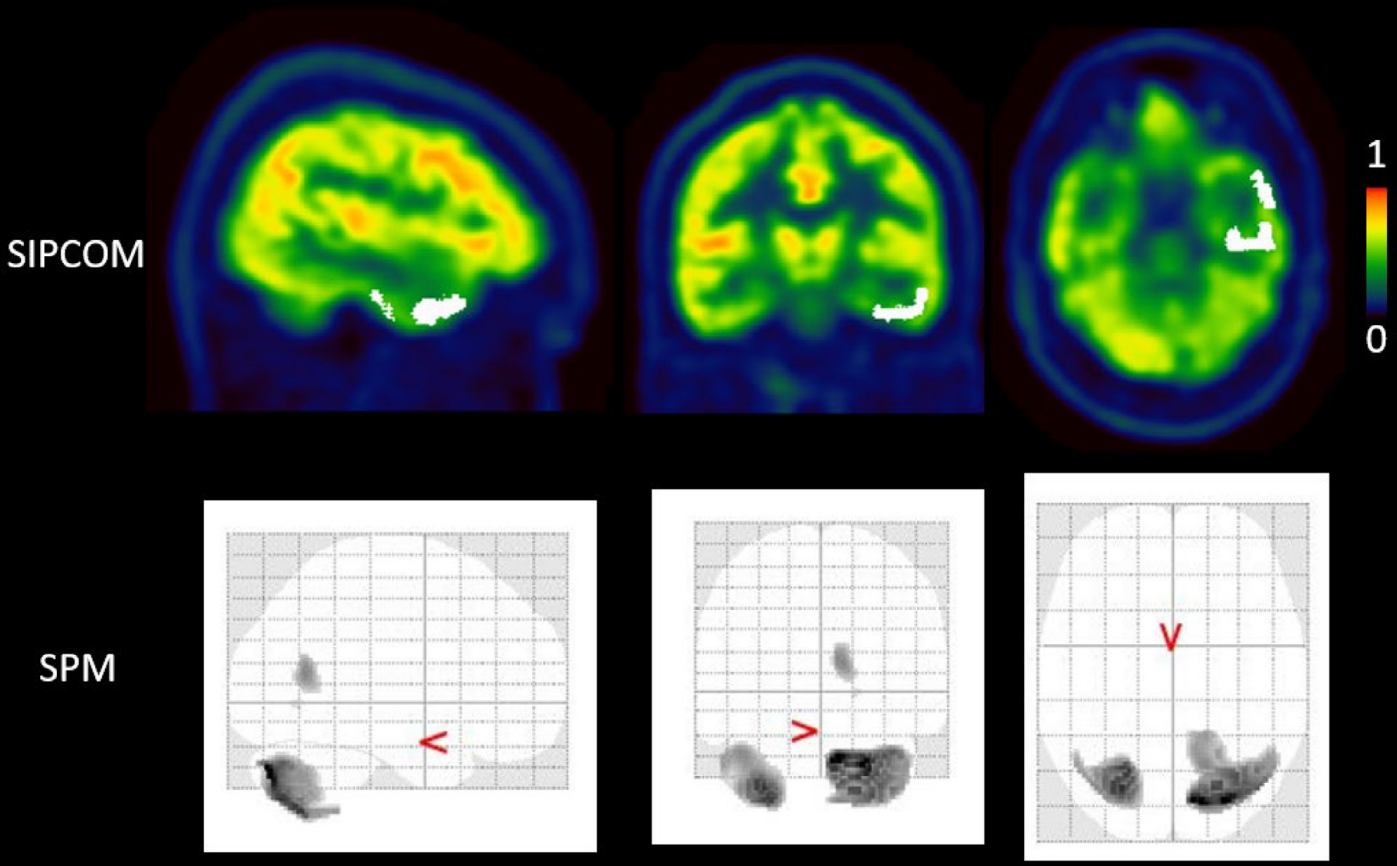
A case (patient #6) with discordant results for Subtraction Interictal PET Co‐registered to MRI (SIPCOM) and statistical parametric mapping (SPM). The first row displays the [^18^F]FDG PET superimposed with an abnormal left temporal cluster defined using SIPCOM. The second row displays the maximum intensity projection result of the SPM analysis for the same patient, showing no cluster of hypometabolism in the left temporal lobe.
